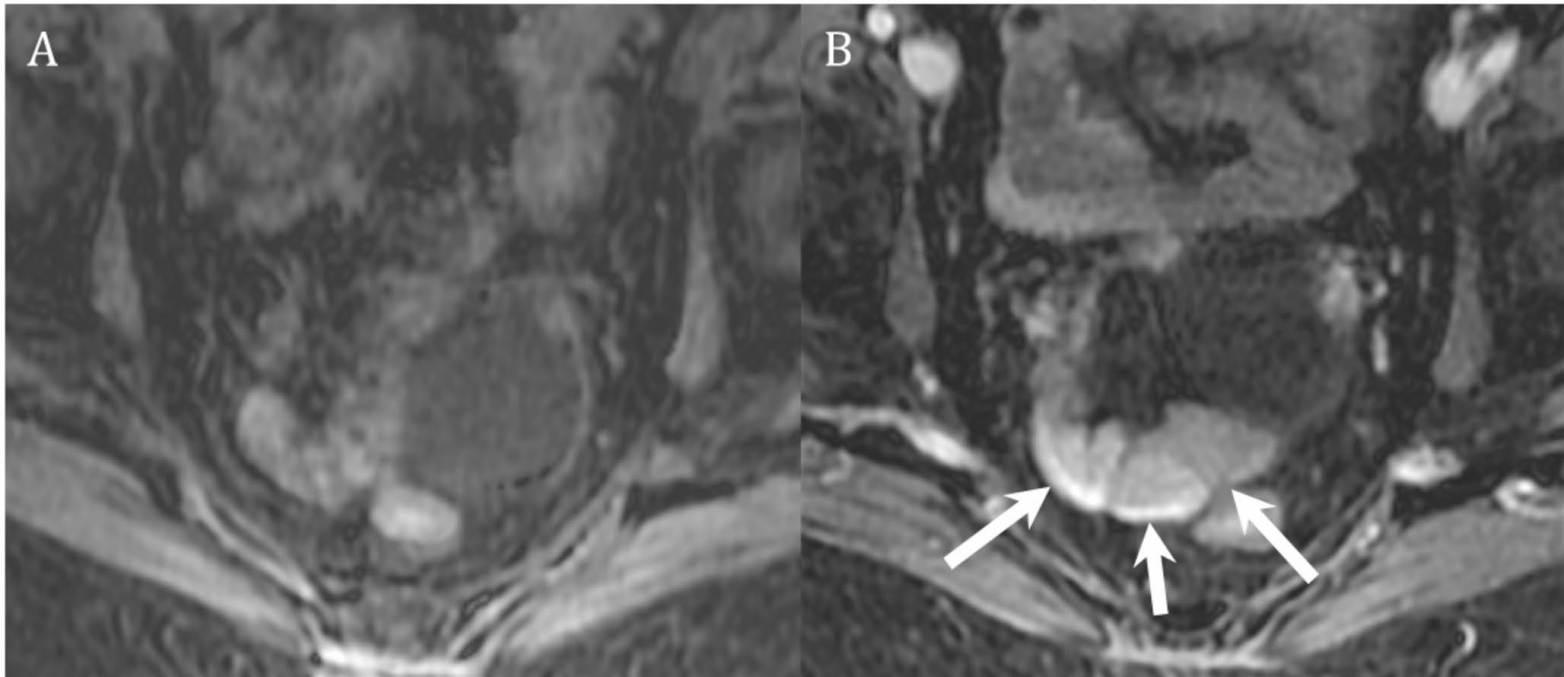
79 year old female presenting with recurrent overt obscure GI bleeding. Prior workup included upper and lower endoscopy, and VCE which demonstrated bleeding in the mid-small bowel. Attempted retrograde double balloon endoscopy failed due to tortuosity of the bowel. Axial precontrast (A), 30 min delayed (not shown) and 3 h delayed (B) postcontrast imaging from MRI enhanced with ferumoxytol demonstrates accumulation of contrast in the distal ileum (arrows, B). The patient underwent targeted fluoroscopic angiography which identified and embolized an ileal angioectasia
